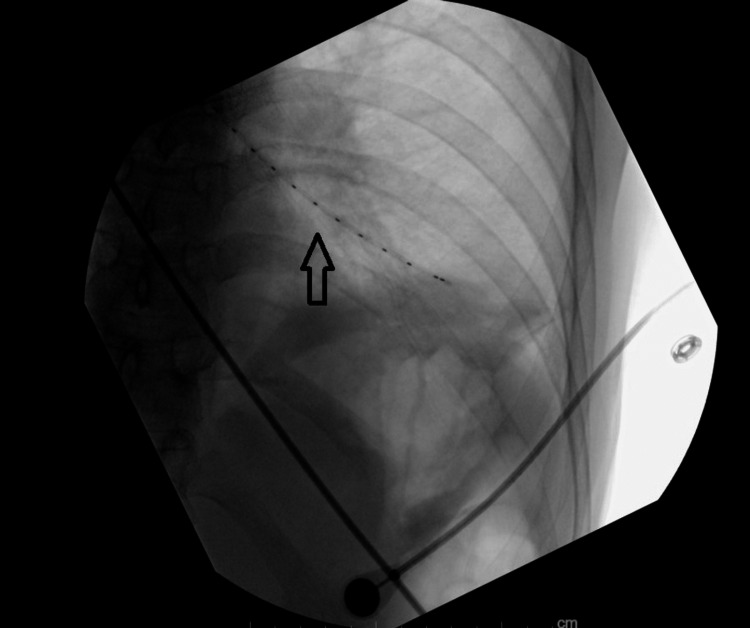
Chest X-Ray Brachytherapy catheter (arrow).
